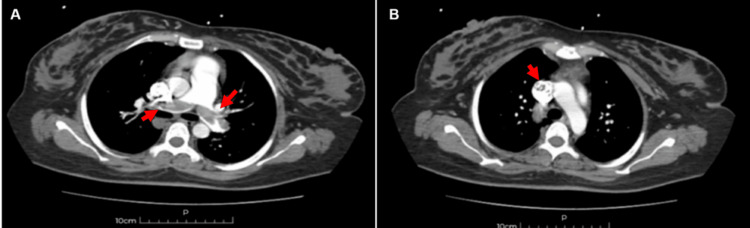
Computed tomography pulmonary angiography (CTPA) (A) CTPA image showing near-complete filling defects (red arrows) involving both main pulmonary artery branches. (B) CTPA image demonstrating a filling defect consistent with thrombus at the level of the superior vena cava (red arrow).
